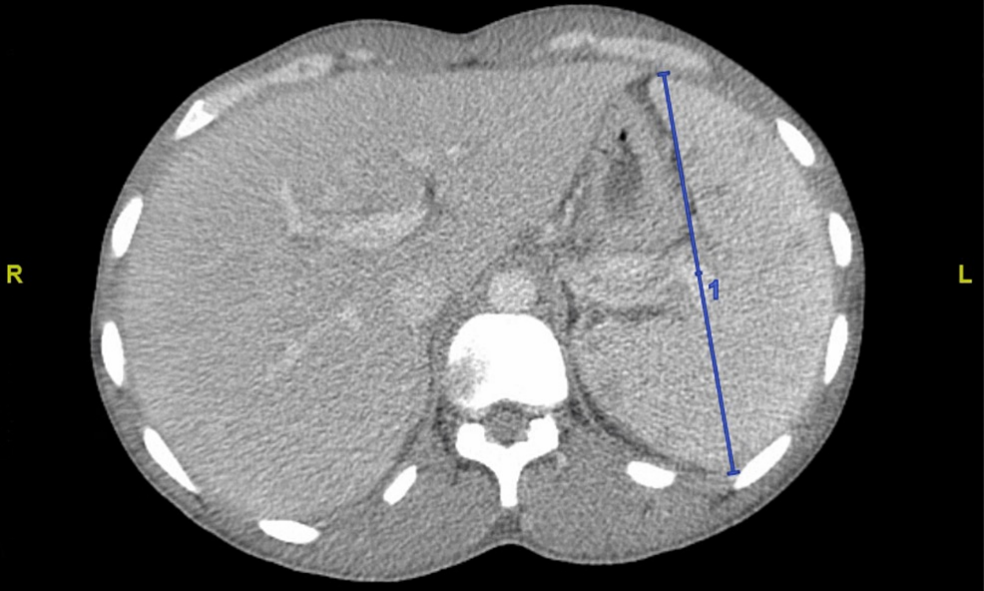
Computed Tomography of the Abdomen Showing Splenomegaly
